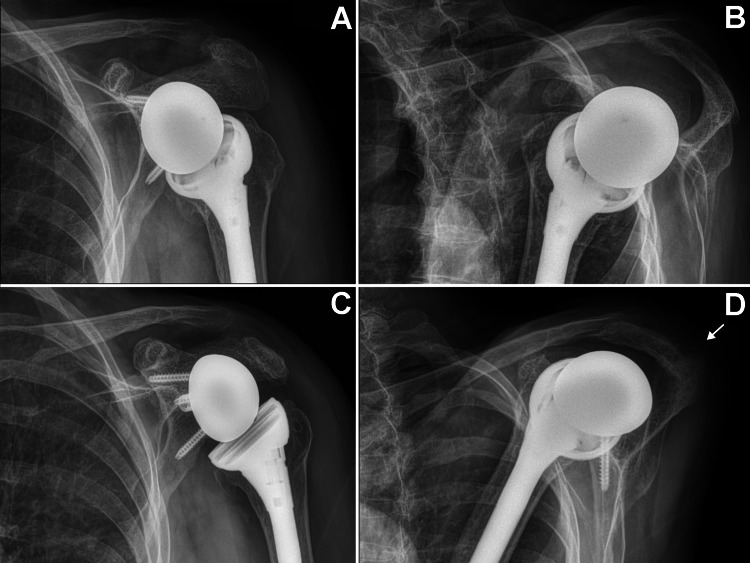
Radiographs taken three weeks (A, B) and four months (C, D) after RTSA A and B: Radiographs taken at three weeks after the RTSA (no complications had occurred). C and D: Radiographs taken at four months after the RTSA showing an angulated acromion stress fracture (arrow) that narrows the subacromial space. There was no pain with palpation of the clavicle.  (A and C) Anterior-posterior views and (B and D) scapula Y views. RTSA: Reverse total shoulder arthroplasty
